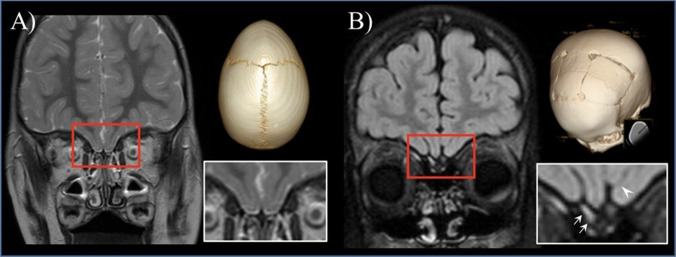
A Coronal T2-image in a 3-year-old boy with trigonocephaly showing bilateral agenesia of the olfactory bulbs and absence of the olfactory sulci. B Coronal FLAIR image in a 6-year-old boy with non-syndromic multisutural craniosynostosis showing left olfactory bulb agenesia with hypoplasia of the ipsilateral olfactory sulcus (arrowhead, note the origin from the midline and its diagonal course). The right olfactory bulb (small arrow) and olfactory sulcus are normal.
